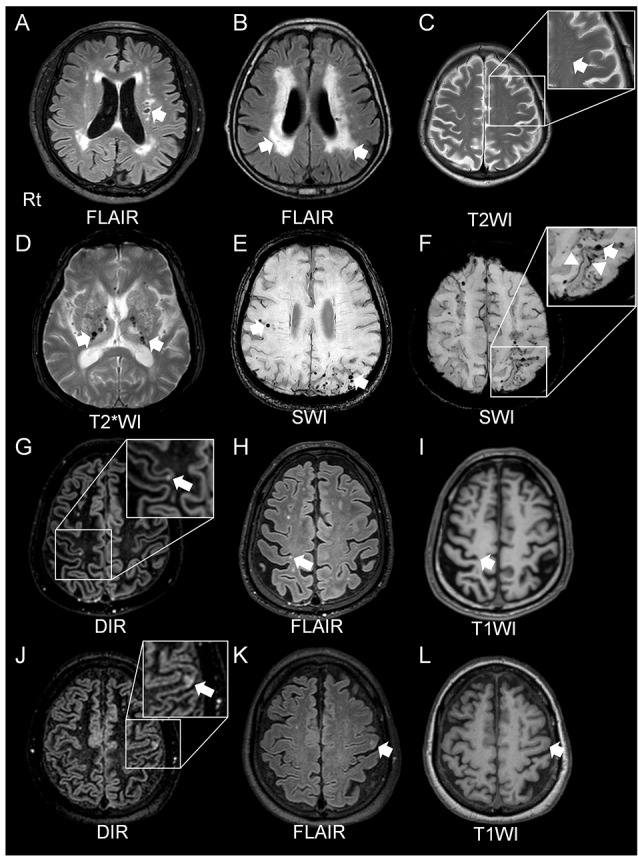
Radiological features of cerebral SVD. A lacunar infarct in the left corona radiata (A) and white matter lesions, assessed by fluid attenuated inversion recovery (FLAIR) imaging, is detected in patients with hypertension (arrows, A,B). The arrow shows enlarged perivascular spaces in T2-weighted (T2WI) magnetic resonance imaging (MRI) in a patient with CAA (C). Cerebral microbleeds (MBs) are classified as two types: deep MBs or lobar MBs. Deep MBs are detected in patients with hypertension on MRI T2*-weighted image (T2*WI; arrows, D). Both lobar MBs and cortical superficial siderosis (cSS) are seen in susceptibility-weighted imaging (SWI) sequences in CAA patients (arrows, E,F, and arrowheads, F). Both double inversion recovery (DIR; G,J) and FLAIR (H,K) imaging can clearly detect the cortical microinfarcts (CMIs). T1*-weighted imaging (T1WI) can also reveal CMIs but less obviously (I,L). CAA and cerebral embolisms can cause CMIs. CMIs from patients with CAAs (G–I) showed that all lesions were localized within cortical structures, with a size of <5 mm (Ishikawa et al., 2020). CMIs caused by embolisms involved subcortical areas, and the size of the lesions was ≥5 mm (Ishikawa et al., 2020).
